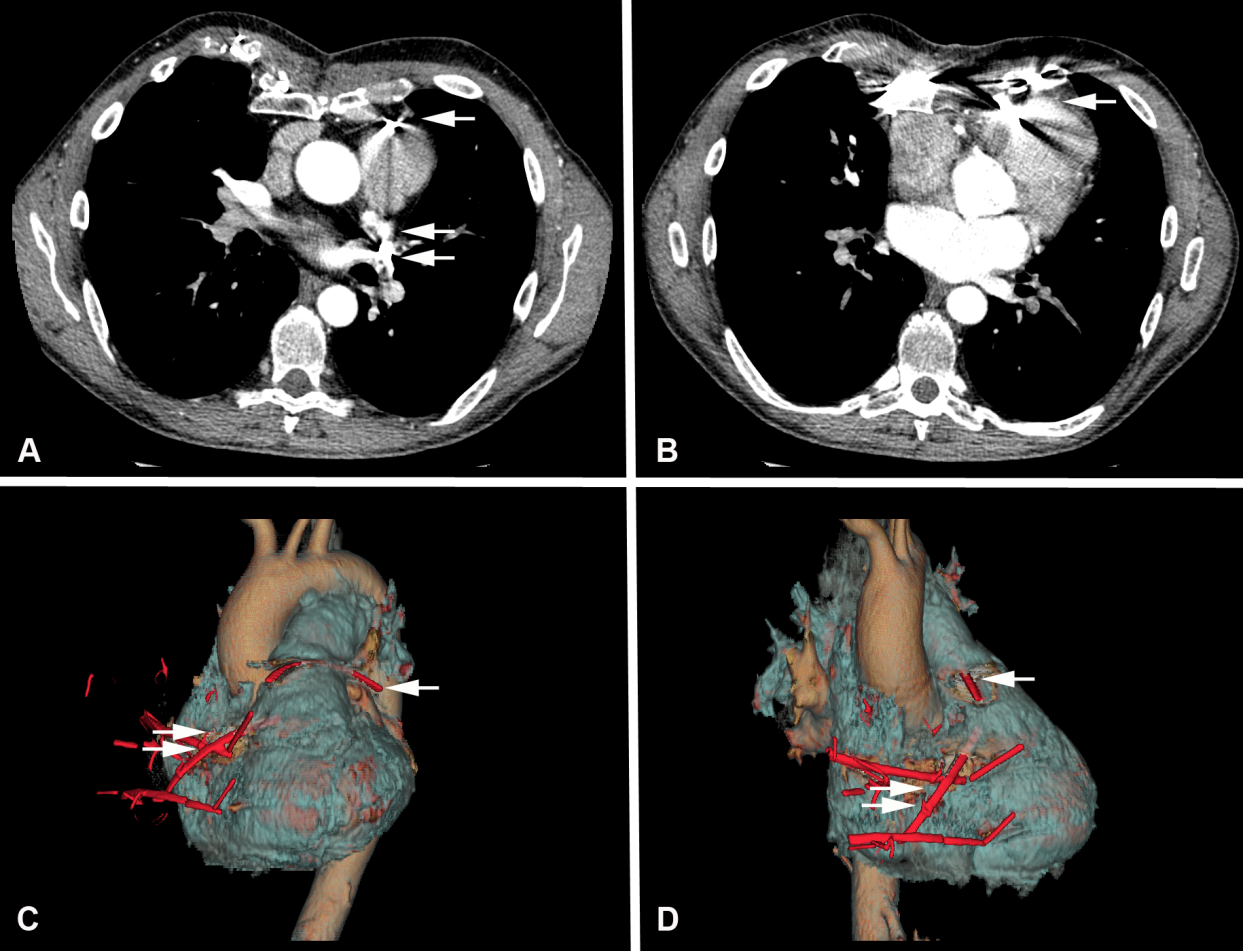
The preoperative CT scan and 3-D reconstruction. A: CT scan showed that the dorsal strut seemed to lie over the bifurcation of pulmonary trunk (single arrow). Its dorsal tip seemed to have perforated the left upper lobe bronchus (double arrow). B: The ventral strut lay behind the sternum. According to the traditional CT scan images, it was not apparent whether the struts perforated into the right ventricle or RVOT due to metal artifact. C and D: The following 3-D reconstruction of CT scan images revealed intracardiac migration of the two struts. The single arrow marked the dorsal strut, which perforated RVOT. The double arrow marked the ventral strut, which perforated into the right ventricle.
